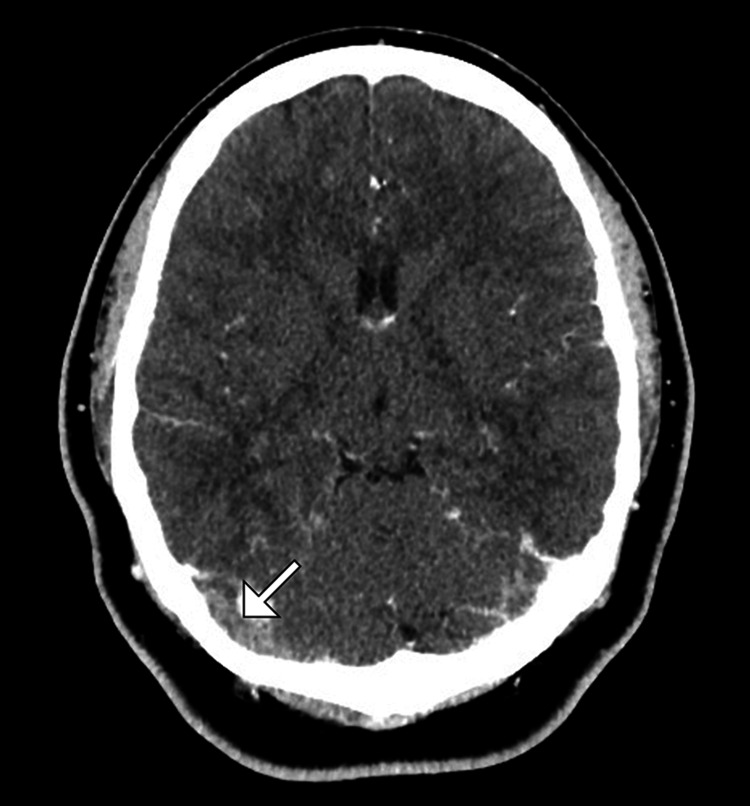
Axial computed tomography venogram showing a filling defect (arrow) along the right transverse sinus, confirming the presence of thrombosis
